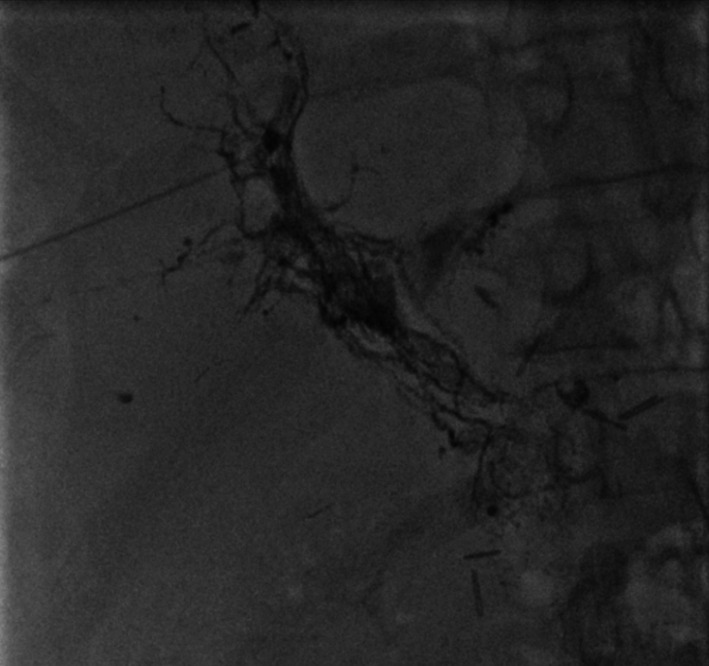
Computed tomography after percutaneous transhepatic lymphangiography and embolization. Image showing accumulation of Lipiodol and *n*-butyl-2-cyanoacylate from the hepatic hilum to the hepatic lymphatic vessels (circle) and disappearance of ascites
